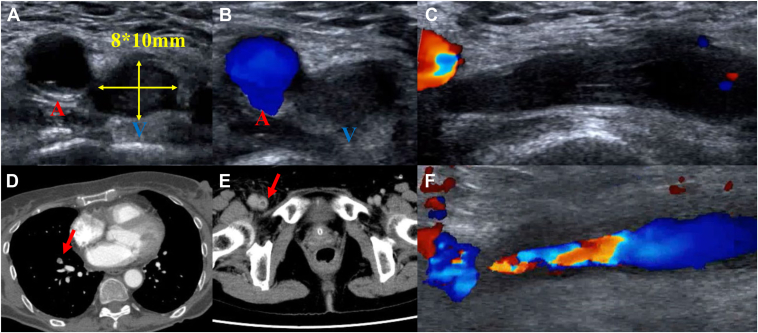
Vascular ultrasound in (A–C) demonstrates occlusion of the right femoral vein. The left vessel corresponds to the right femoral artery and the right vessel to the right femoral vein (A–B). Contrast-enhanced computed tomography revealed a contrast defect in a peripheral branch of the right lower pulmonary artery (D) and an occlusion of the right femoral vein (E). A follow-up lower limb venous ultrasound on the fourth hospital day demonstrated recanalization of the lower leg veins, although stenosis remained (F).
